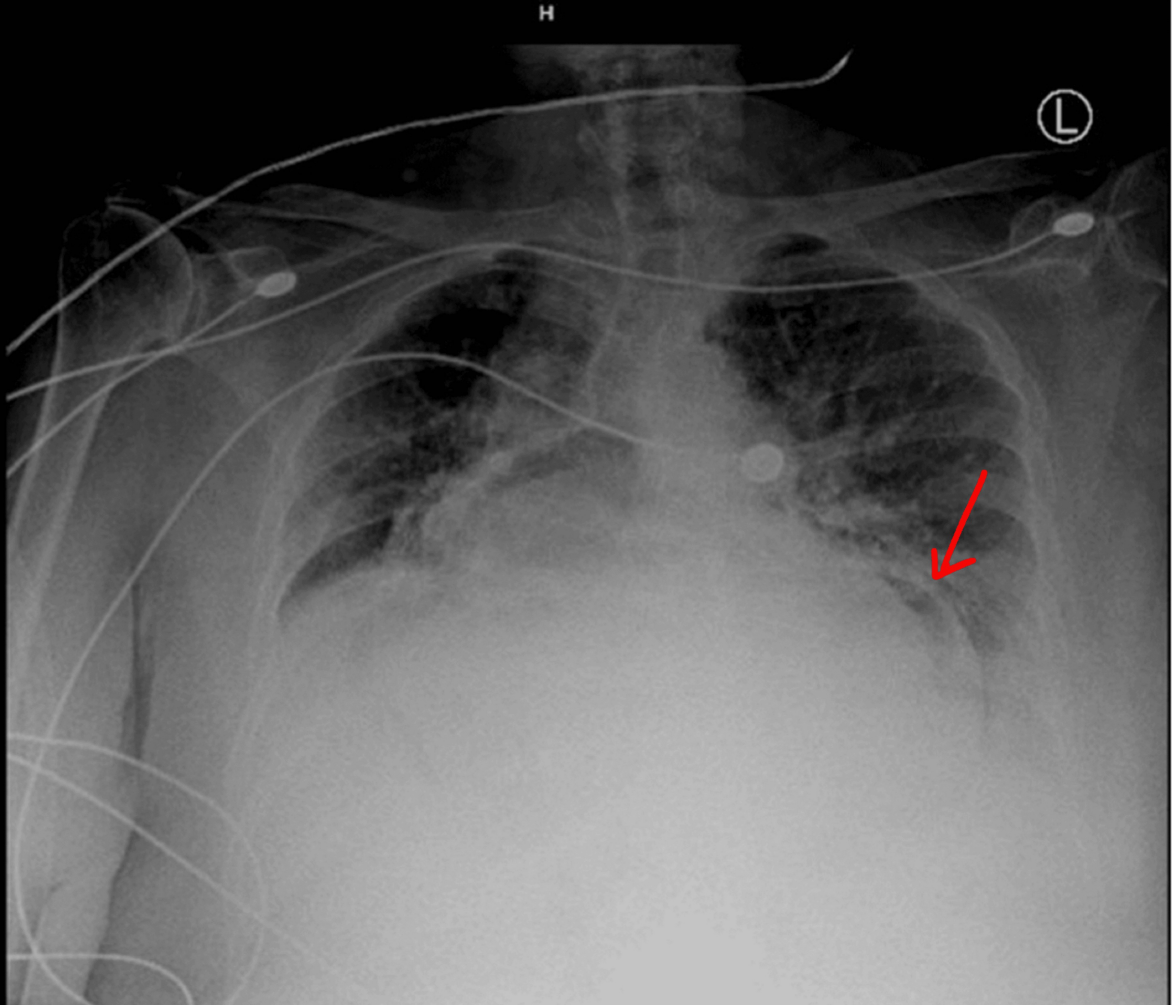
Chest X-ray revealing pulmonary vascular congestion and bibasilar effusions The presence of air on the left side of the cardiac silhouette is highlighted by a red arrow, denoting the stomach. The positioning of the stomach within the thoracic cavity is indicative of a hiatal hernia.
